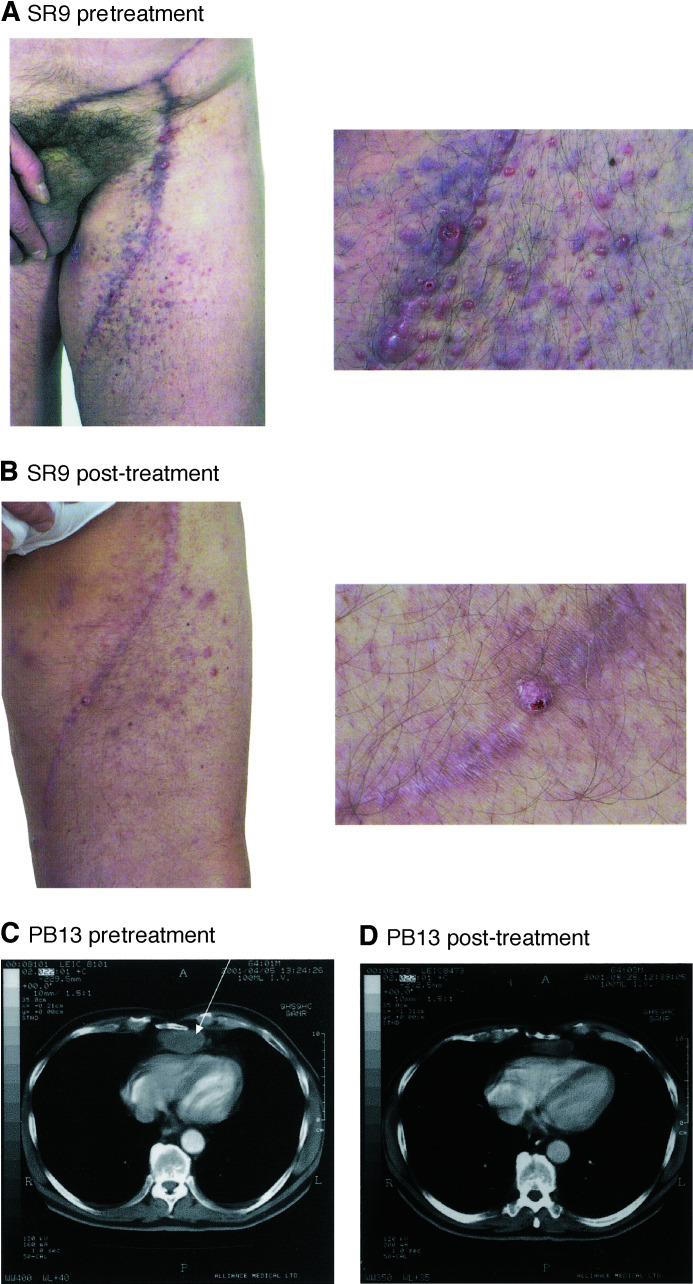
Evidence of clinical response during CC-5013 treatment. (A) Patient SR9 exhibited extensive nodular cutaneous disease over his left thigh. Following treatment (B) lesions became flattened and attenuated. (C) Patient PB13 shows mediastinal mass (arrowed) on CT scan which is reduced (D) after treatment.
